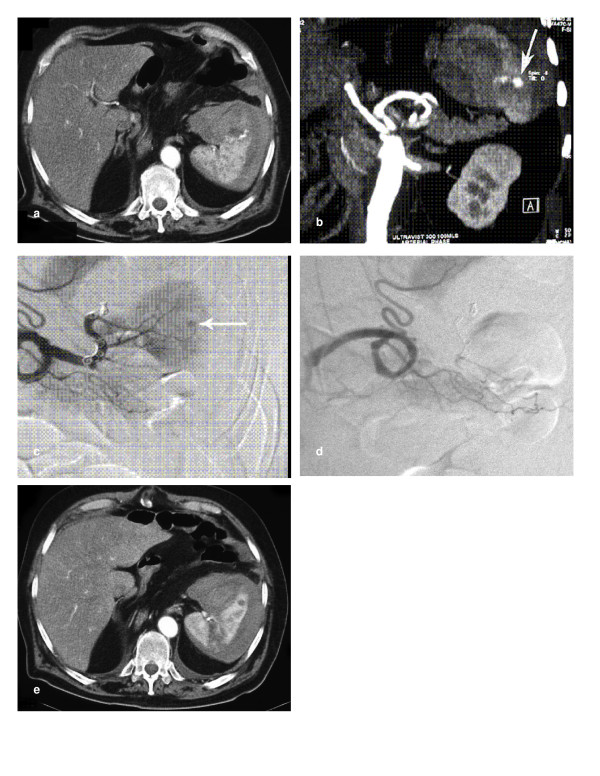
a) Axial CT of a 73 year old man with iatrogenic splenic injury following chest drain insertion. An active bleeding point in the spleen (arrow) with surrounding haematoma was demonstrated. b) Coronal CT reconstruction showing a tortuous splenic artery and bleeding point (arrow). These allowed optimal catheter choice for arteriography. c) A Tracker-18 microcatheter system with a Fasdasher 0.014 in wire (Boston Scientific, Maple Grove, MN, USA) were used to achieve access distally within the splenic circulation. After several unsuccessful attempts at superselective catheterisation of the branch supplying the bleeding point, 4 platinum Vortex-18 diamond-shaped coils (Boston Scientific) were deployed sequentially in the main splenic artery distal to the dorsal pancreatic branch. 2 initial coils migrated past the required branch and there is ongoing bleeding from the spleen (arrow). d) The next 2 coils achieved occlusion of the main splenic artery with preservation of branches to the dorsal pancreas and upper pole of the spleen. e) Axial CT at 1 week showed a small splenic infarct where the initial coils had migrated distally. Arterial supply to the spleen was preserved with some flow through the main splenic artery coils.
